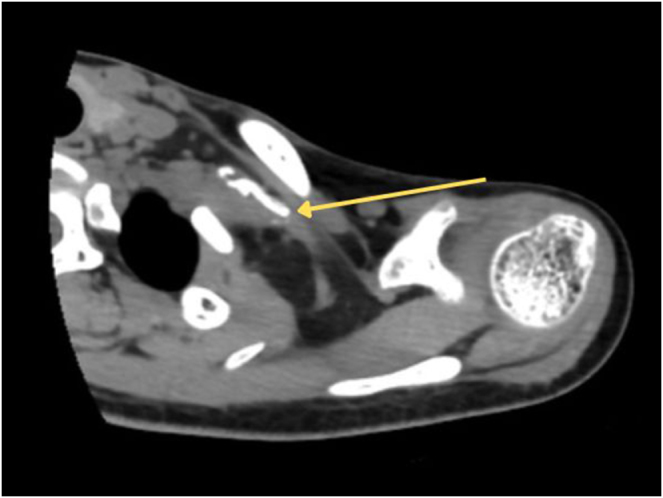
Preoperative axial shoulder CT scan showing the *double clavicle* sign, an ossified mass of brachial plexus (yellow arrow) located between the clavicle and the first rib.
